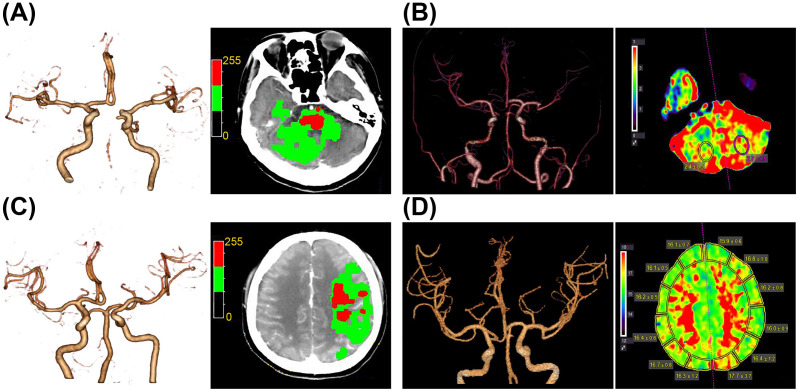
Imaging manifestations of BAO and ACS patient before and after CTP guided EVT treatment. (A) Preoperative CTA showed occlusion of the basilar artery and CTP showed low perfusion in the basal artery supply area, with the red area indicating the core infarct zone. (B) Postoperative CTA indicated recanalization of the basilar artery and CTP indicated the recovery of blood flow in the basal artery supply area. (C) Preoperative CTA showed occlusion of the upper trunk of the M1 segment of the left middle cerebral artery and CTP showed hypoperfusion in the left middle cerebral artery supply area, with the red area indicating the core infarct zone. (D) Postoperative CTA showed recanalization of the left middle cerebral artery and CTP indicated the recovery of blood flow in the left cerebral blood supply area.
